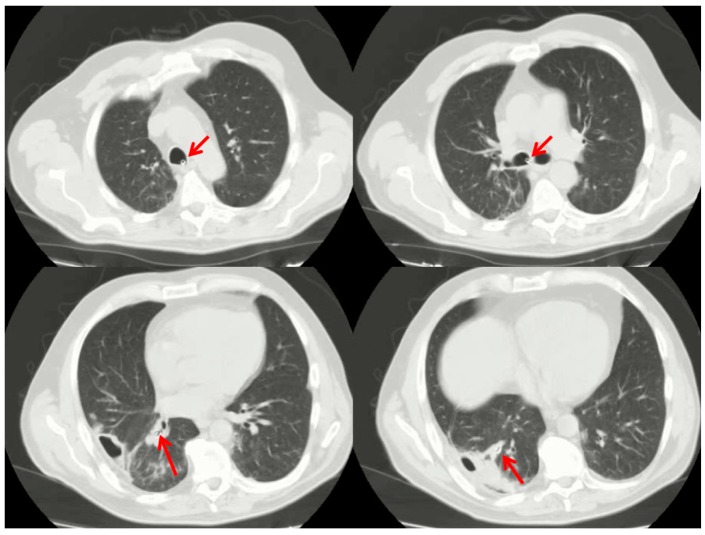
Chest computed tomography scan confirmed the prompt position of catheter (red arrows).
